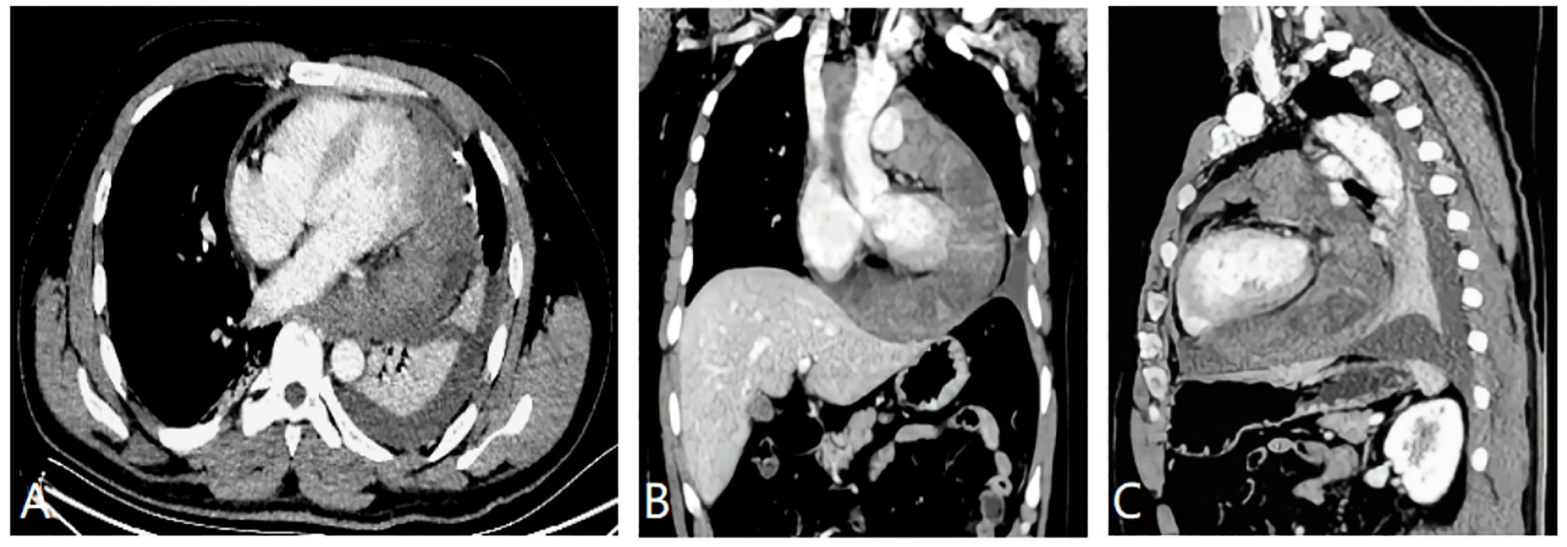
(A) Mildly enhanced axial CT image showing the large heterogenous left pericardial mass. There was a slight pericardial effusion. (B) Enhanced CT image of the coronal reconstruction showing the tumor circumscribing the ascending aorta, the superior vena cava, and the pulmonary artery. (C) CT image of the sagittal reconstruction showing the mass invading the pericardium, which presented uneven thickening andnodular changes.
